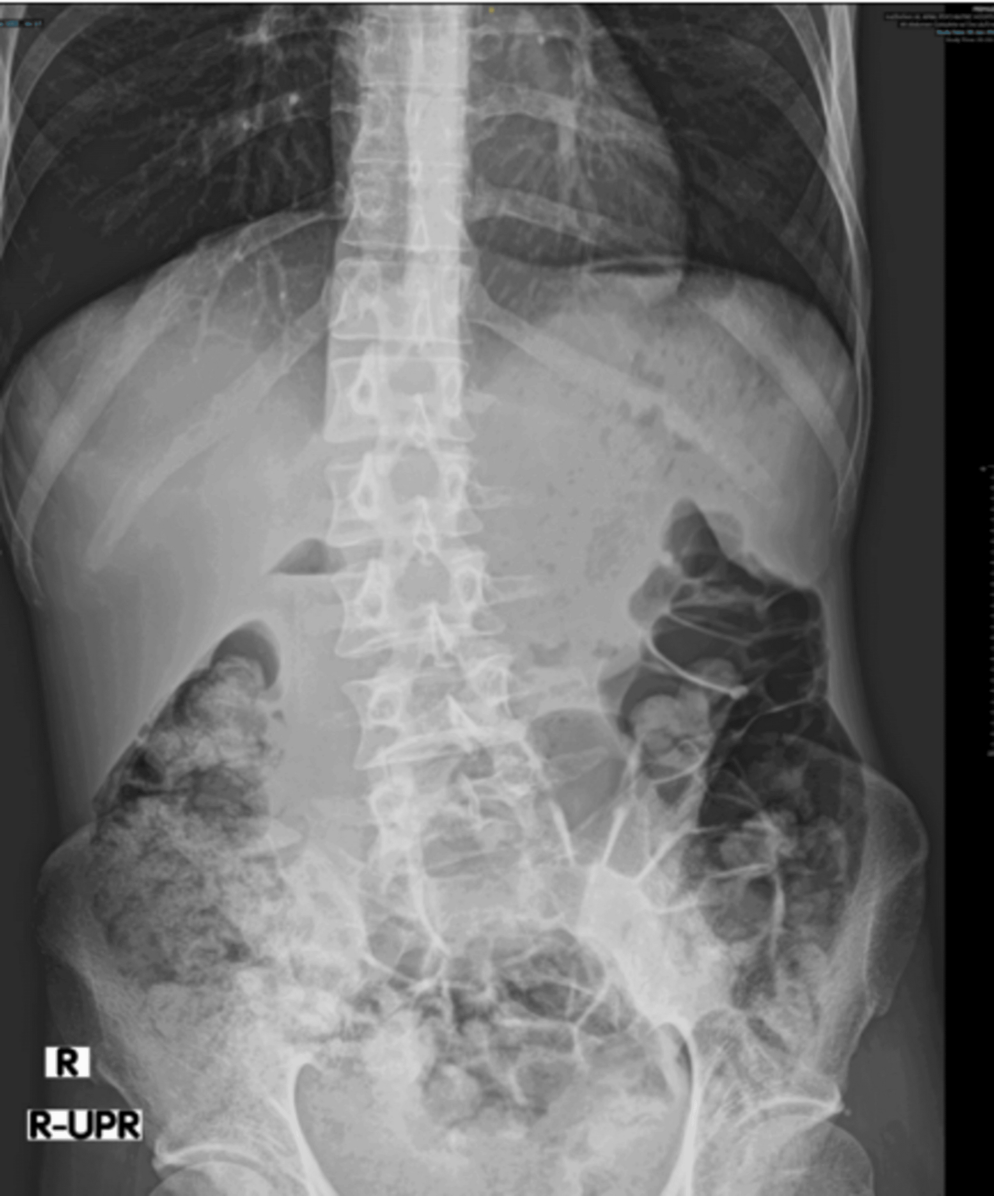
Abdominal X-ray revealing gaseous and fecal matter loading of colonic loops
